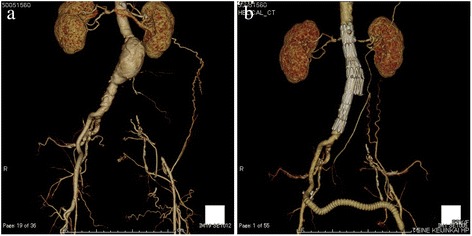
Computed tomography shows preoperative (a) and postoperative (b) conditions. Both internal iliac arteries are preserved
